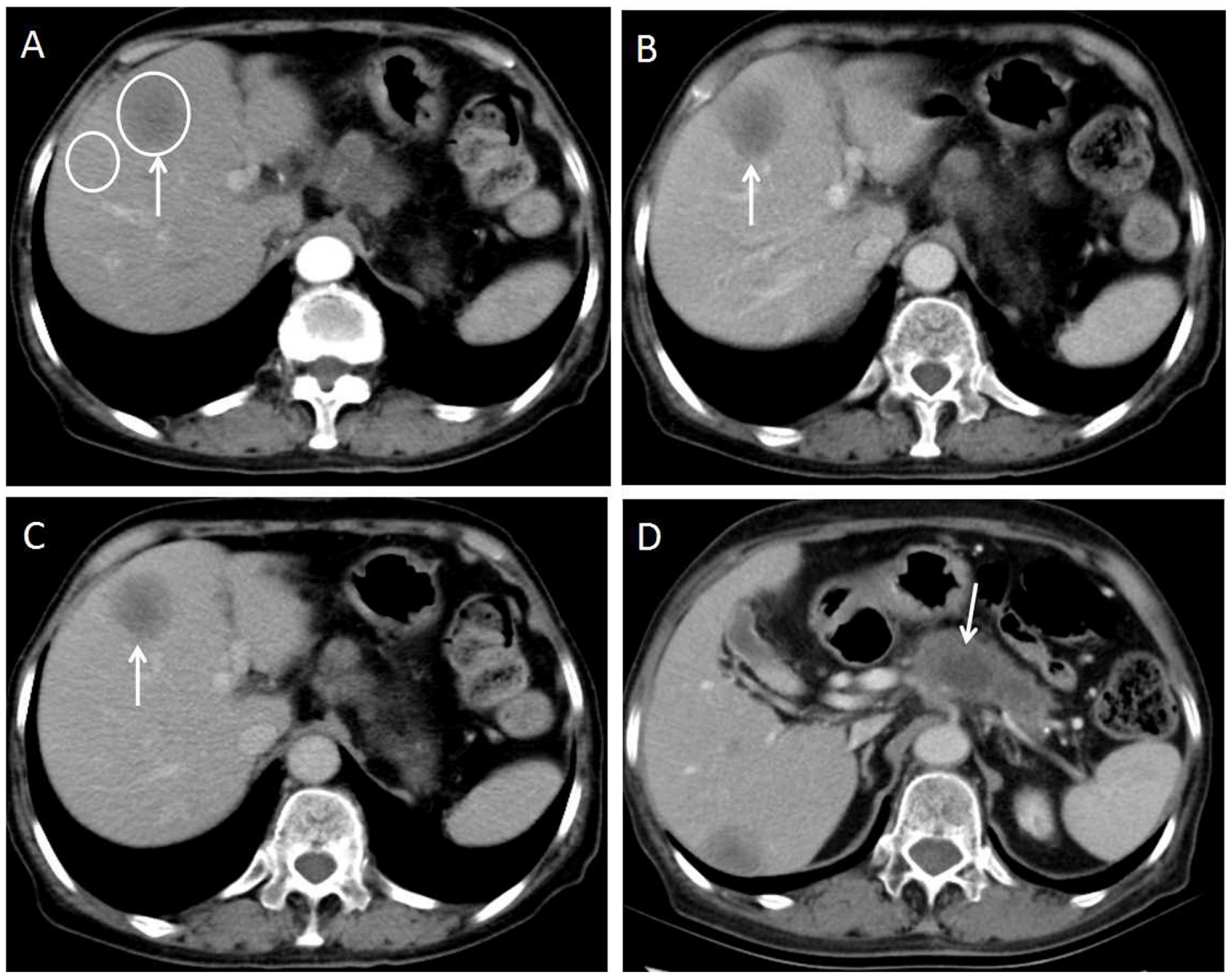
70-year-old woman with moderately-poorly differentiated pancreatic adenocarcinoma (A) Axial CT image on the hepatic arterial phase shows a hypo enhanced liver metastasis in the left lobe of the liver(arrow). Two oval shaped ROIs were placed on the largest lesion and adjacent normal liver. The metastasis-to-liver ratios on hepatic artery phase and portal venous phase were 70.7% and 60.2% respectively. (B and C) Axial CT image on the portal venous phase and equilibrium phase show the lesion keeps hypo enhancement that meets the plateau enhancement pattern(arrows). (D) Axial CT image on the portal venous phase shows hypo enhanced primary tumor in the pancreas(arrow).
